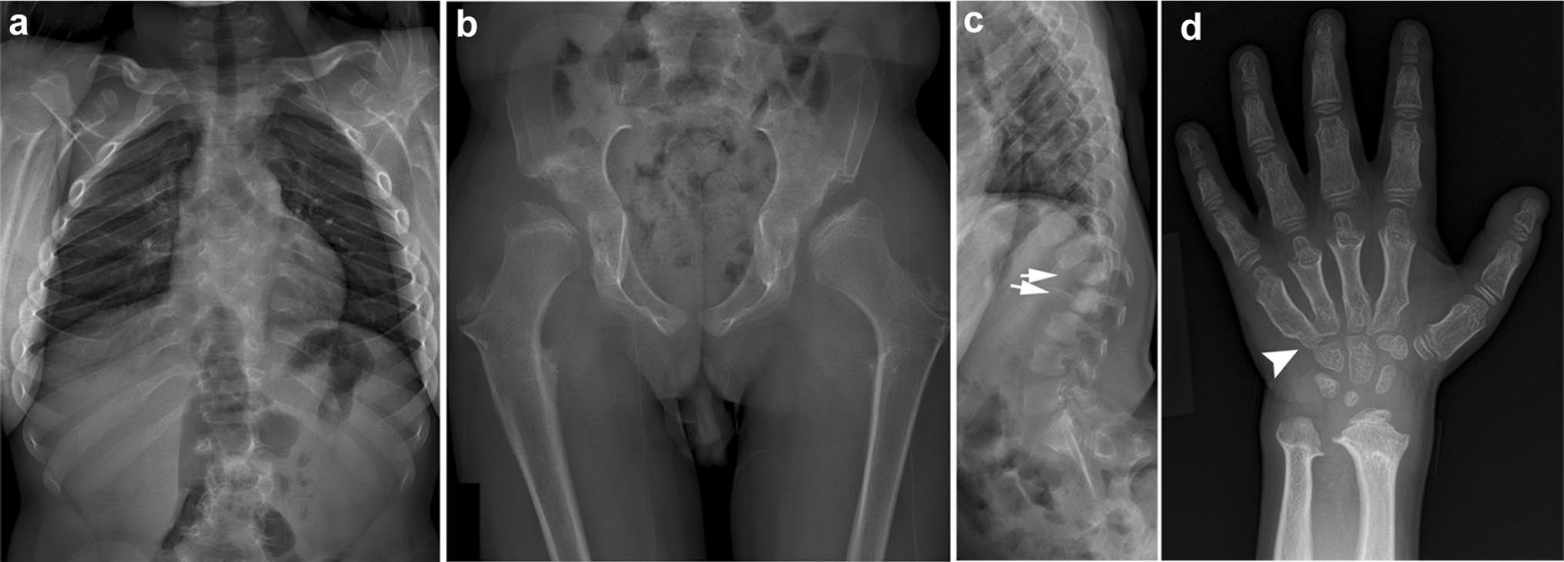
a–d An 11-year-old boy with Hurler syndrome. Radiographs show findings of dysostosis multiplex including a thick clavicles, paddle-shaped (or oar-shaped) broad ribs, b comma-shaped ilia, diaphyseal broadening and metaphyseal constriction of the long bones, c hook-shaped vertebral bodies (arrows) with posterior scalloping, d proximal pointing of the metacarpals (arrowhead), and bullet-shaped phalanges
